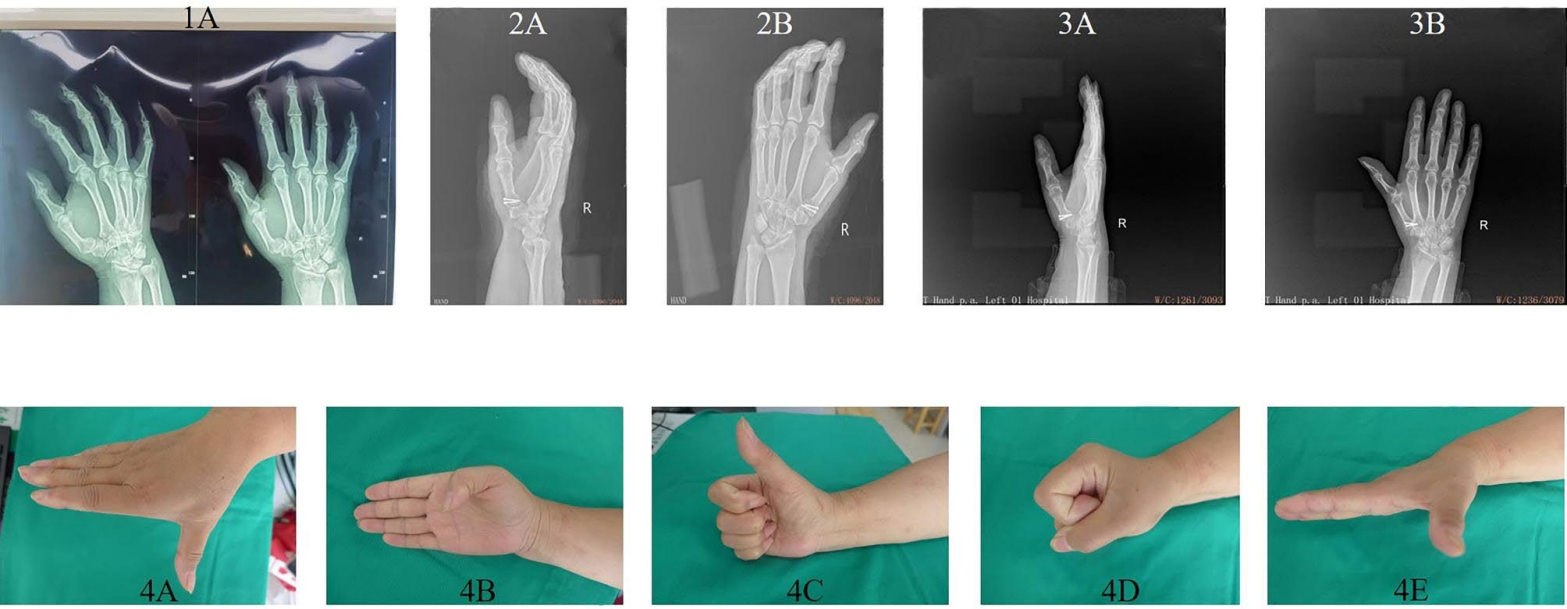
Representative clinical treatment course of a patients treated with hollow screw fixation. The patient suffered first metacarpal bone base fracture because of fall damage. (1 A) X-ray examination before operation showed first metacarpal bone base fracture. (2 A and 2B) X-ray examination after hollow screw fixation showed that the first carpometacarpal joint surface was restored, and the first carpometacarpal joint was in position. The fixation was fixed for 4 weeks after operation, and functional exercise was started after 4 weeks. (3 A and 3B) X-ray examination two months after operation showed that the fracture healed. (4 A-E) The function recovered well at 8 months after operation, with no joint movement pain and limitation
